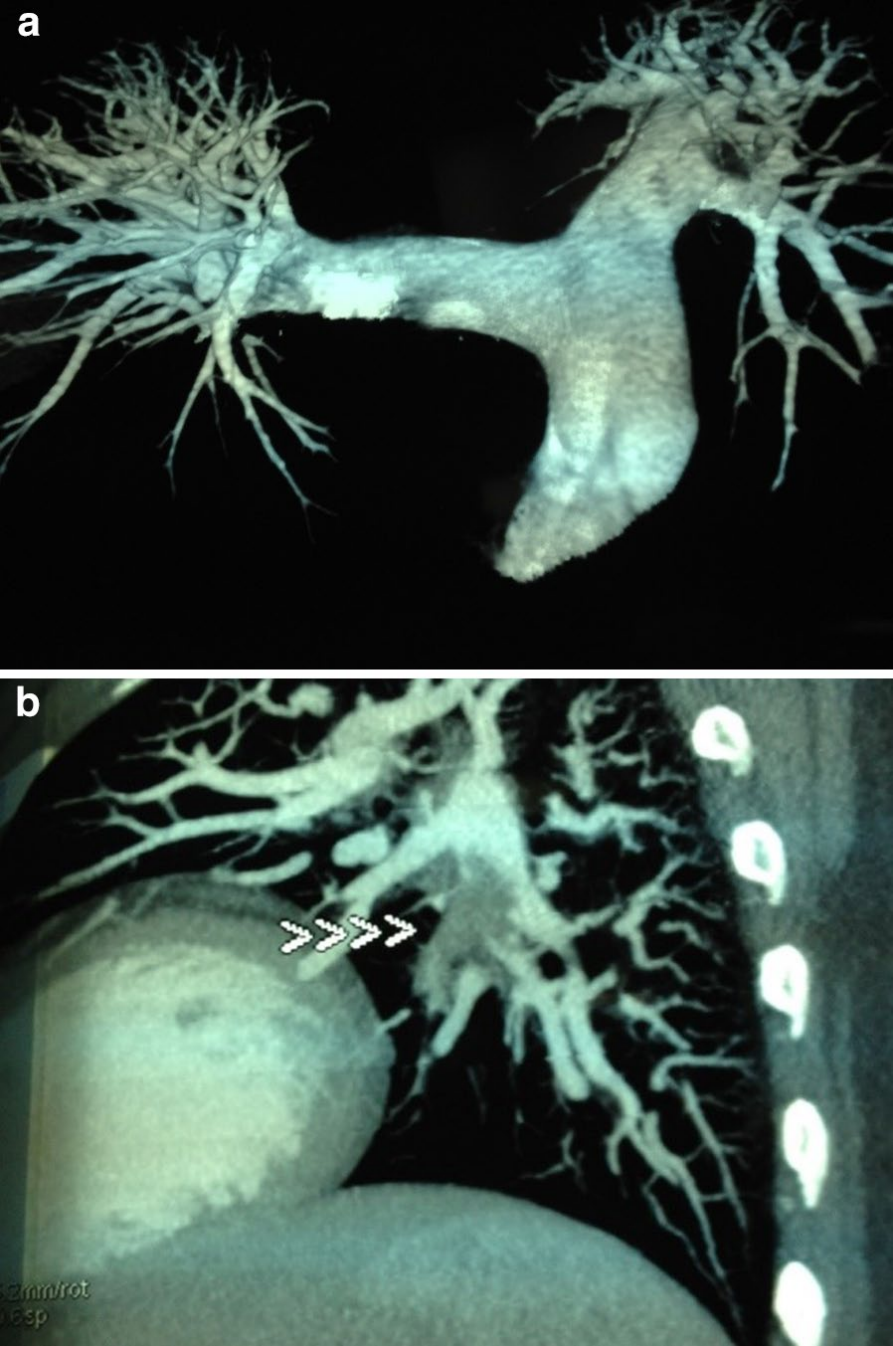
a CT pulmonary angiography showing thrombus. b CT pulmonary angiogram shows filling defects in descending branch of left pulmonary artery (*arrow heads* in b) extending up to segmental arteries, suggesting thrombus
